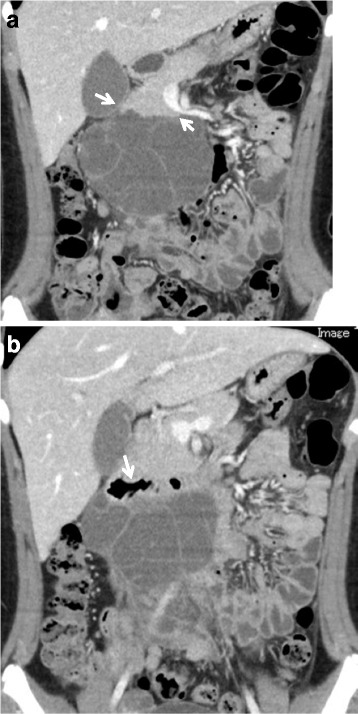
Contrast CT revealed a well-circumscribed multicystic mass measuring 9.5 × 5.8 × 10.0 cm in the retroperitoneal region. The mass, filled with serous fluid without hemorrhage or abscess, was suspected to adhering and stretching the pancreas head (a, *arrow*: pancreas head) by the beak sign. The tumor was suspected to adhering the duodenum (b, *arrow*: duodenum)
